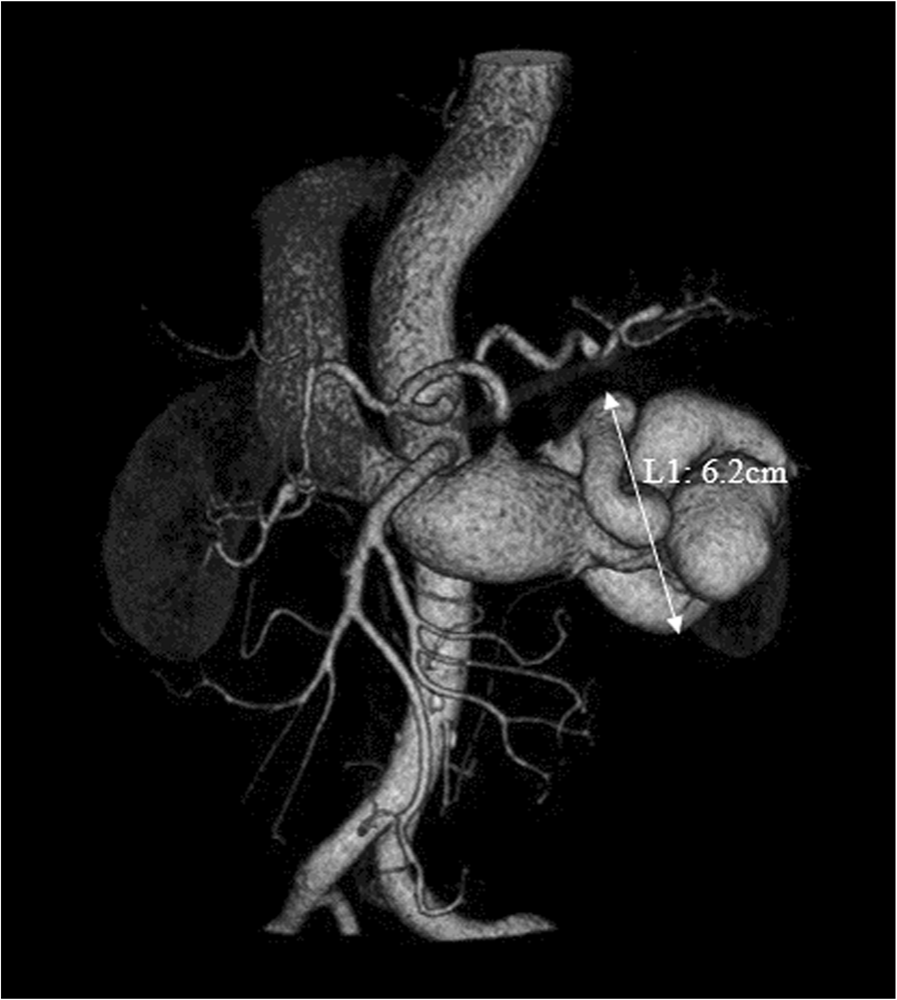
Pre-operative three-dimensional computed tomography (3D CT) reconstruction demonstrated a large left RAVF. Malformed blood vessels (L1), 6.2 cm diameter, could be clearly observed adjacent to the renal hilum
